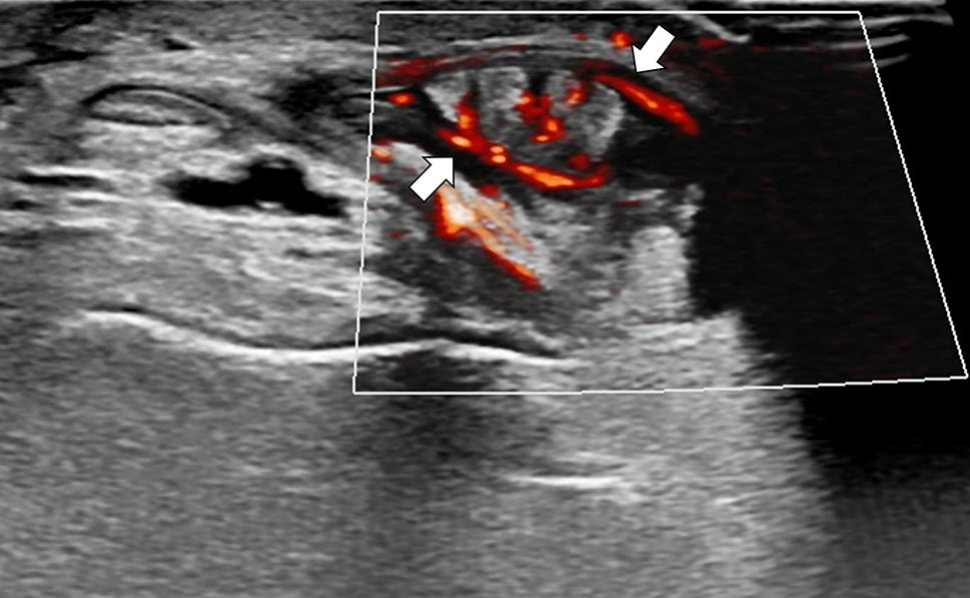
Increased blood flow within the tenosynovium revealed in ultrasonography of the left ankle joint
